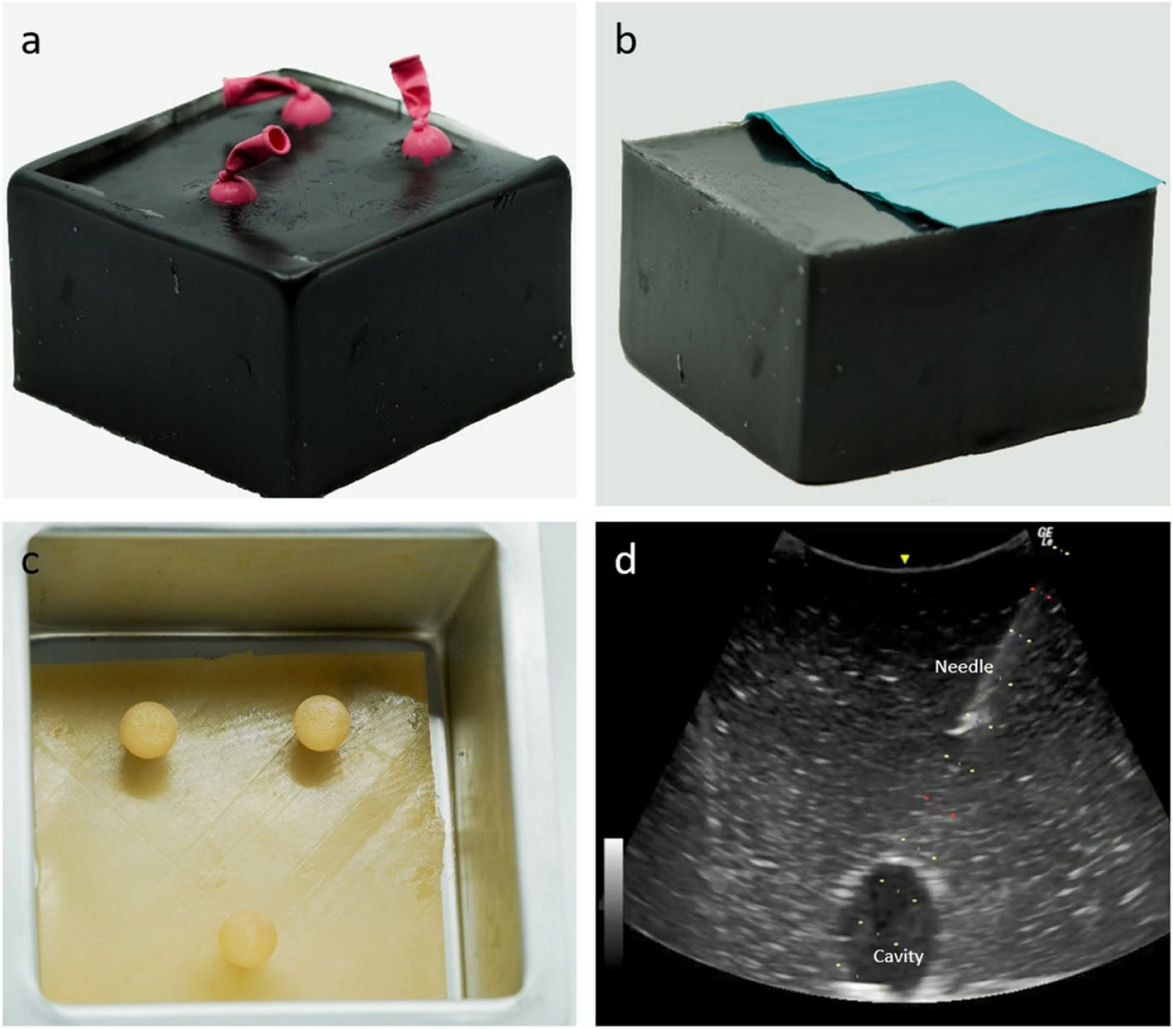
Ultrasound-guided aspiration trainer. a Positions of waterfilled cavities*. b Cover used to encourage different probe movements*. c Silicon-mat in aluminum mold used to shape the ballistic gel*. d Example of ultrasound output
